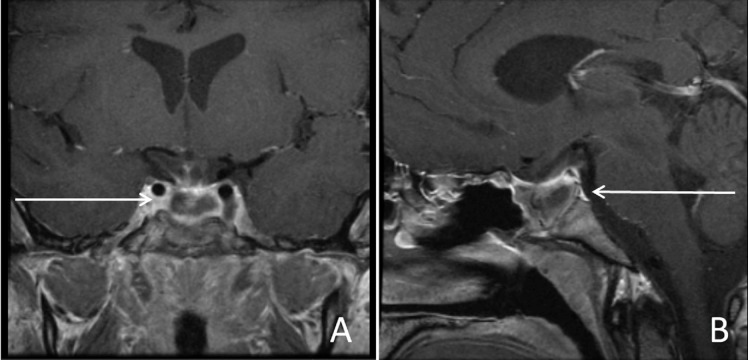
Postoperative magnetic resonance imaging (MRI) with contrast demonstrating minimal residual disease, coronal (A) and sagittal (B).
